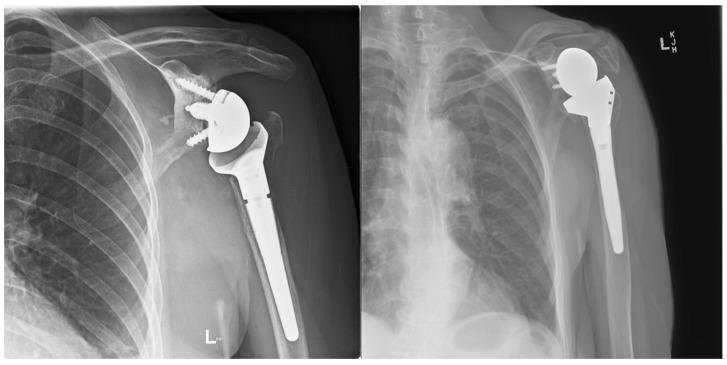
Preoperative X-rays showing proximal stress shielding and bony resorption above the level of the pectoralis major insertion.
